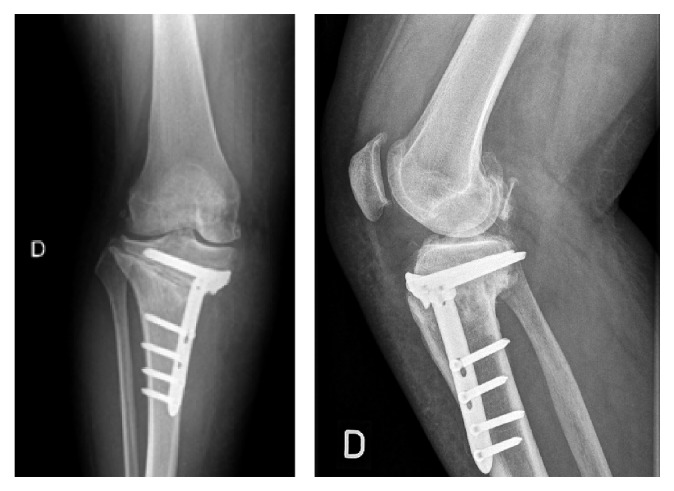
July 2013, 3 years after revision, postoperative X-rays showing increasing signs of osteoarthritis.
